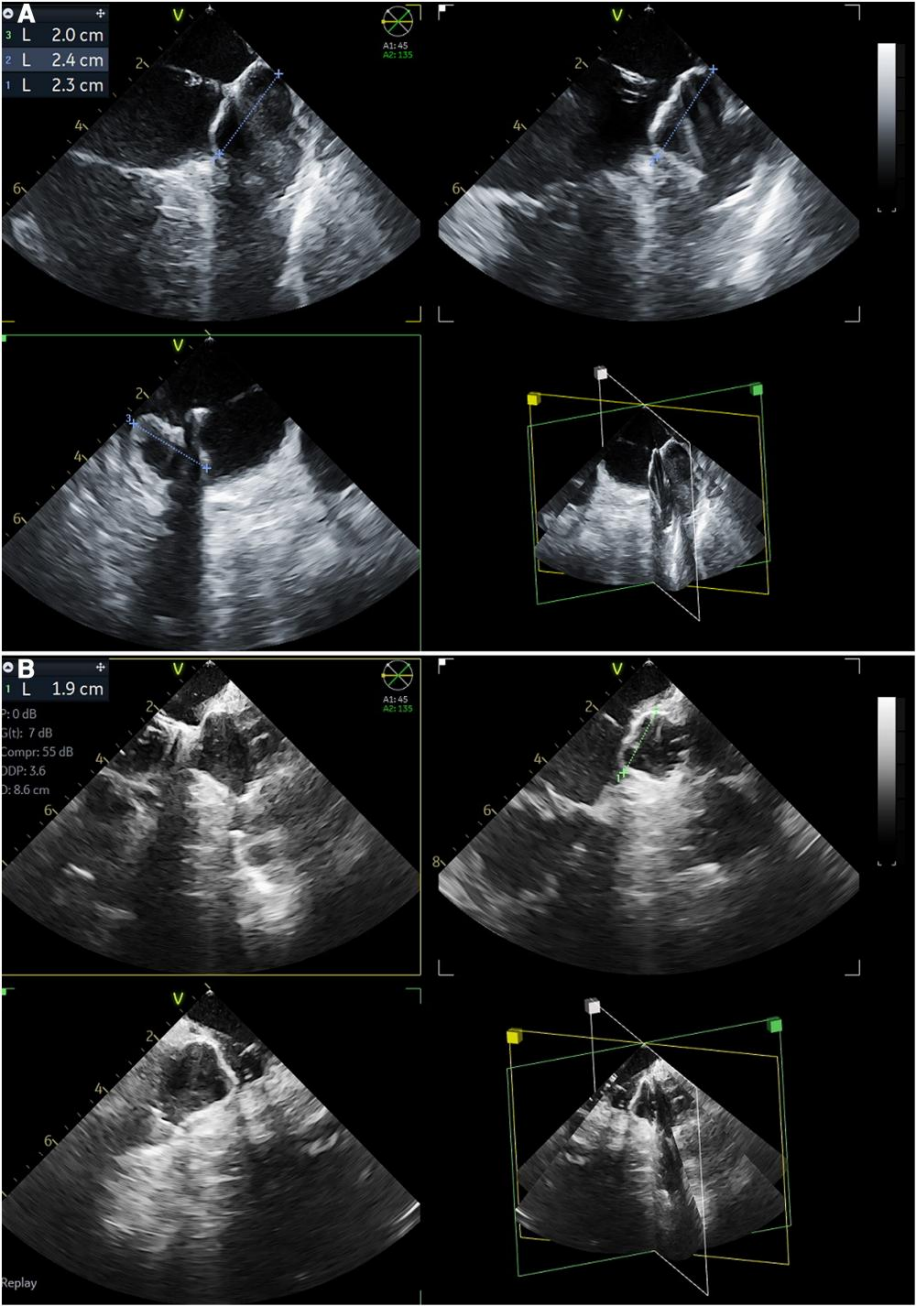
Assessment of adequate device position and compression in two patients (panels *A* and *B*). Watchman deployment and device sizing from 3D-ICE multiplanar reconstruction (45°–90°–135°) to assess stability, compression, and appendage sealing (presence of residual leaks). ICE, intracardiac echocardiography.
